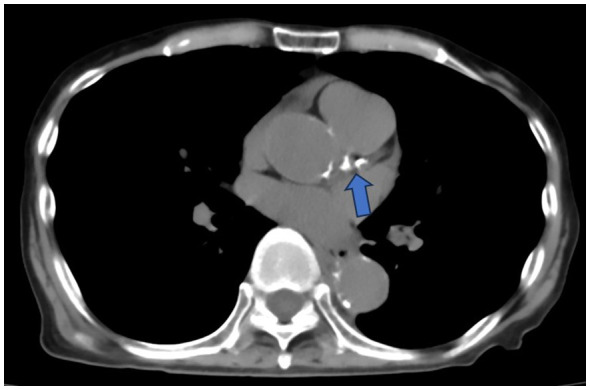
Non-contrast, nongated chest CT scan from an 88-year-old male with COPD and osteoporosis.
